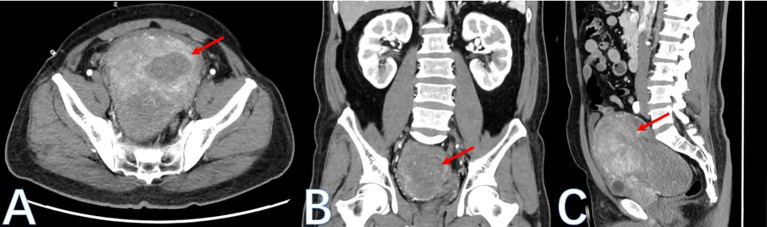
Computed tomography urography (CTU) images of the giant pelvic solitary fibrous tumor (SFT) in Case 1. (A) Axial contrast-enhanced CTU image showing a large heterogeneous soft-tissue mass measuring 17.5 × 14.5 × 11.8 cm with marked heterogeneous enhancement and a prominent superficial venous plexus. (B) Coronal contrast-enhanced CTU image demonstrating significant compression and displacement of the bladder, seminal vesicles, and rectum by the mass. (C) Sagittal contrast-enhanced CTU image confirming the well-circumscribed border of the giant tumor and its anatomical relationship with adjacent pelvic structures. Red arrow indicates the pelvic SFT.
